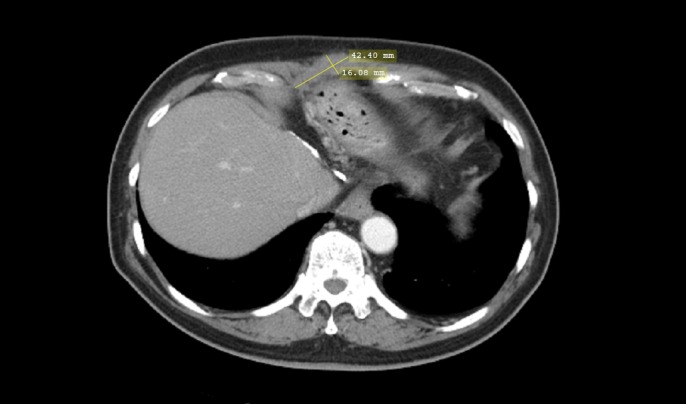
CT Abdomen After Treatment With Pembrolizumab Axial contrast-enhanced CT of the abdomen showing marked interval decrease in the size of the recurrent HCC measuring 2.2 x 1.6 cm.
